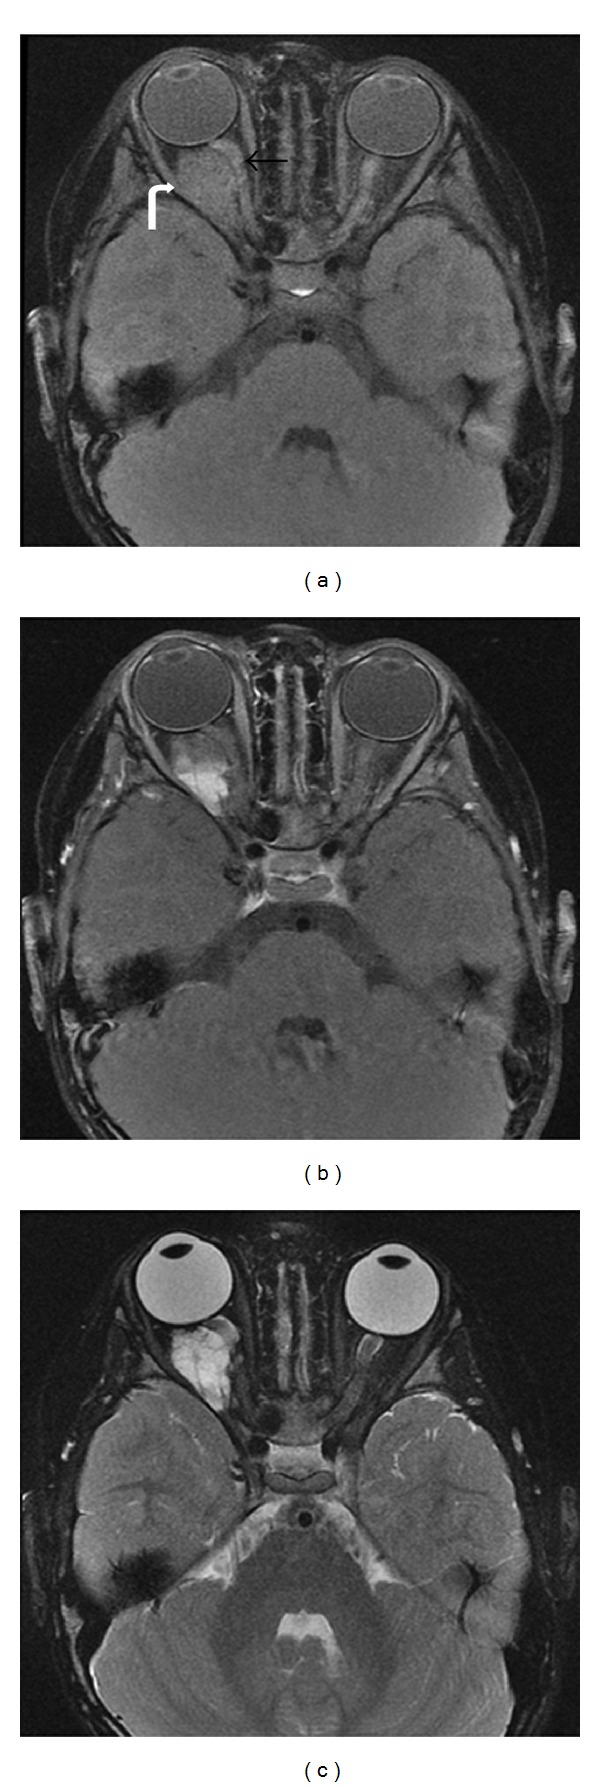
Patient with blue rubber bleb nevus presenting with right proptosis. Precontrast T1-weighted axial images with fat saturation (a) show a well-defined, lobulated, and intraconal mass (curved arrow) displacing the right optic nerve (black arrow). Postcontrast T1-weighted fat-saturated axial image (b) shows patchy, irregular, and enhancement. On T2-weighted fat-saturated image (c), the mass is hyperintense with linear low-intensity areas, likely flow voids. These findings were consistent with a hemangioma.
